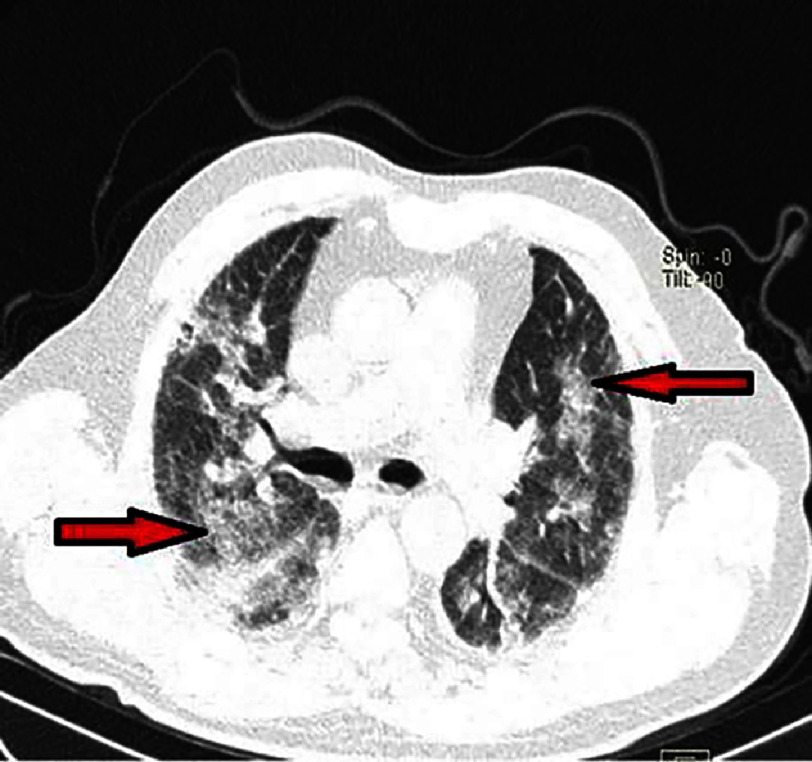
Chest CT scan showing diffuse bilateral ground-glass opacities on a lung parenchyma window (Patient #1).
